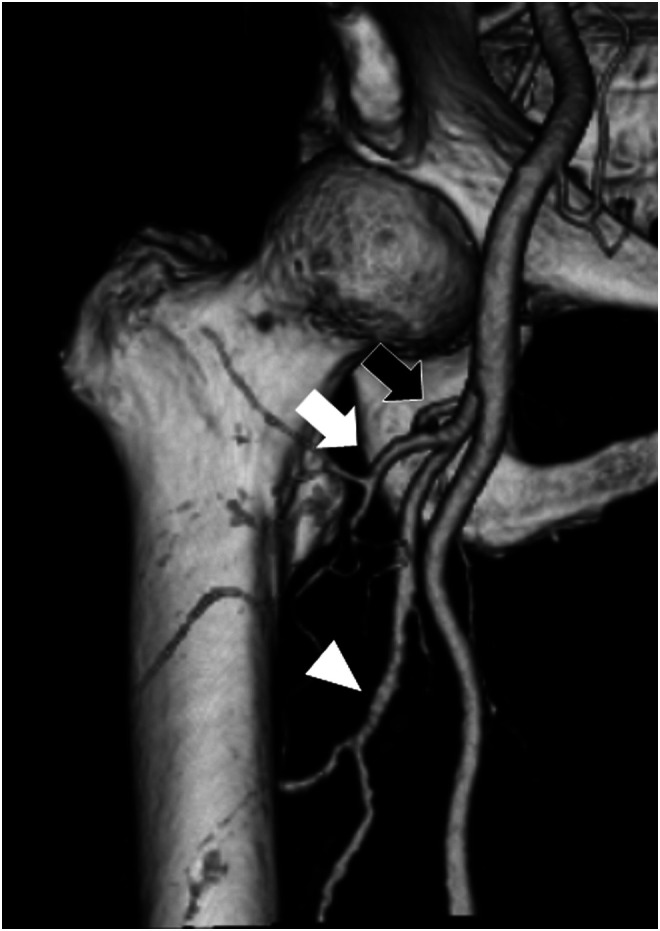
Three-dimensional reconstructed computed tomography image showing arteries that supplied blood to the proximal femur. Lateral femoral circumflex artery (white arrow) runs in front of the femur. MFCA (black arrow) delivered blood to the femoral neck and the head from the posterior aspect of the femur. DFA (white triangle) runs along the femoral shaft.
